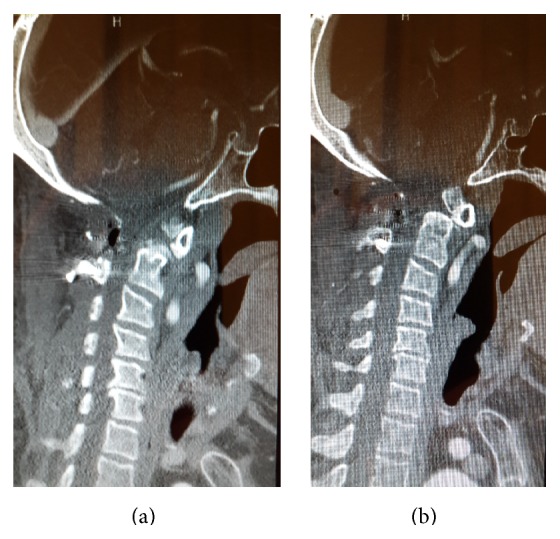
Sagittal reconstructed CT plus angiography, showing optimal alignment, and decompression of cervicomedullary junction achieved with C1 arch laminectomy and limited occipital laminectomy. Note shortness of clivus, low Trochlor Herophili, and low external occipital protuberance and integrity of the basilar artery.
